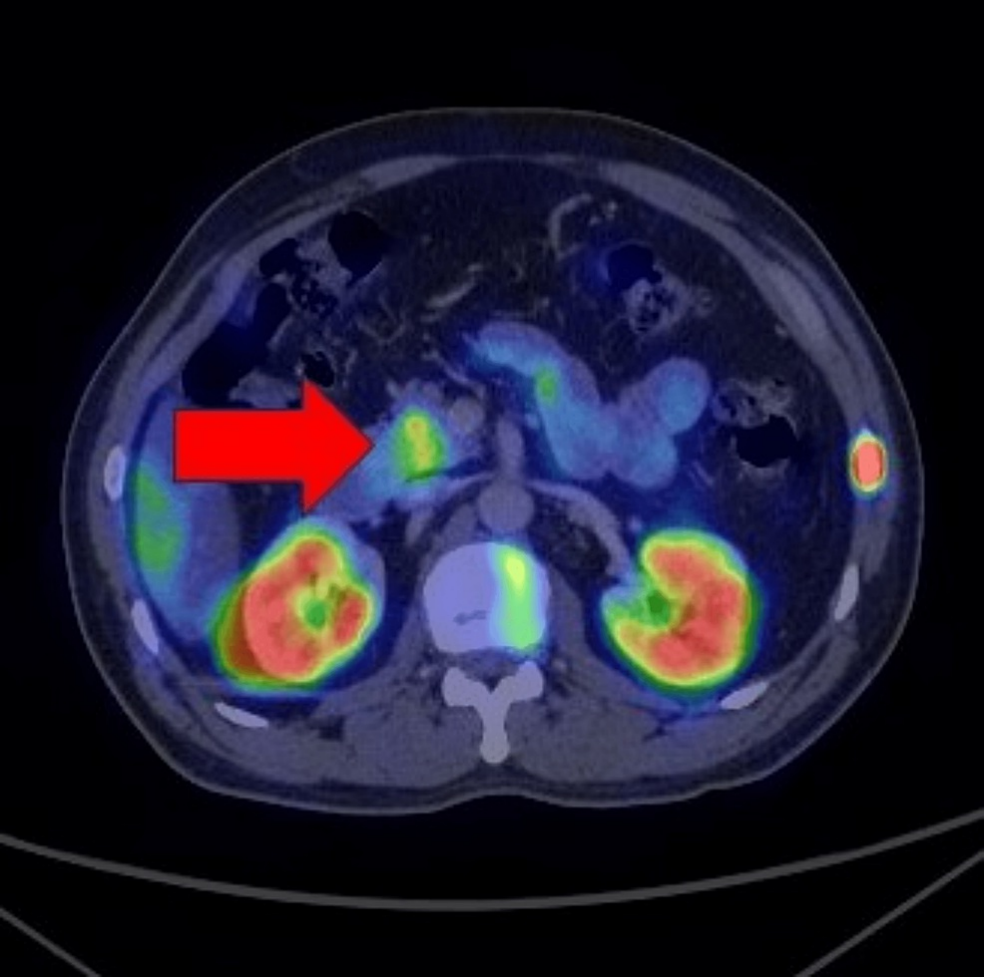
Axial 68Ga-DOTANOC PET-CT image showing the tracer avid pancreatic neuroendocrine tumor (red arrow).
